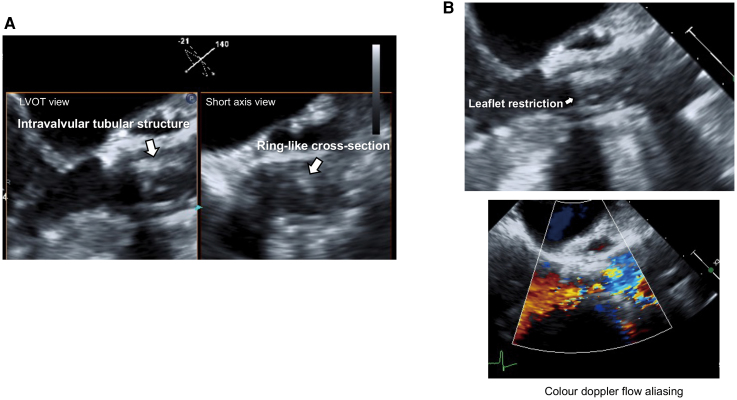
Transoesophageal echocardiography demonstrating acute leaflet opening restriction caused by an intravalvular tubular structure. (*A*) Biplane views showing an intravalvular tubular structure within the self-expanding transcatheter heart valve: tubular appearance in the left ventricular outflow tract (LVOT) view and ring-like in cross-section on the short-axis view (arrows indicate the intravalvular tubular structure). (*B*) Long-axis view demonstrating leaflet restriction (arrow) and colour Doppler showing flow aliasing across the transcatheter heart valve, consistent with significant functional obstruction.
